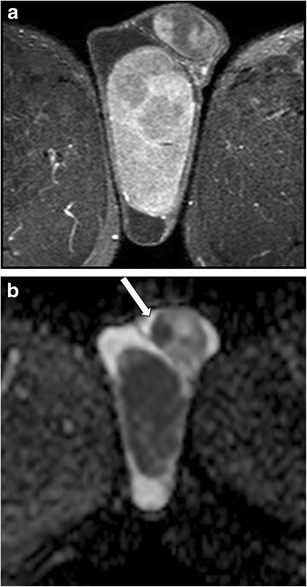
a-b MRI in a case of bilateral seminoma. a Post-contrast T1-weighted SPIR TSE sequence, axial view and b ADC map, demonstrating a large right testicular mass and a smaller left intratesticular one (arrow), with heterogeneous contrast enhancement and restricted diffusion
